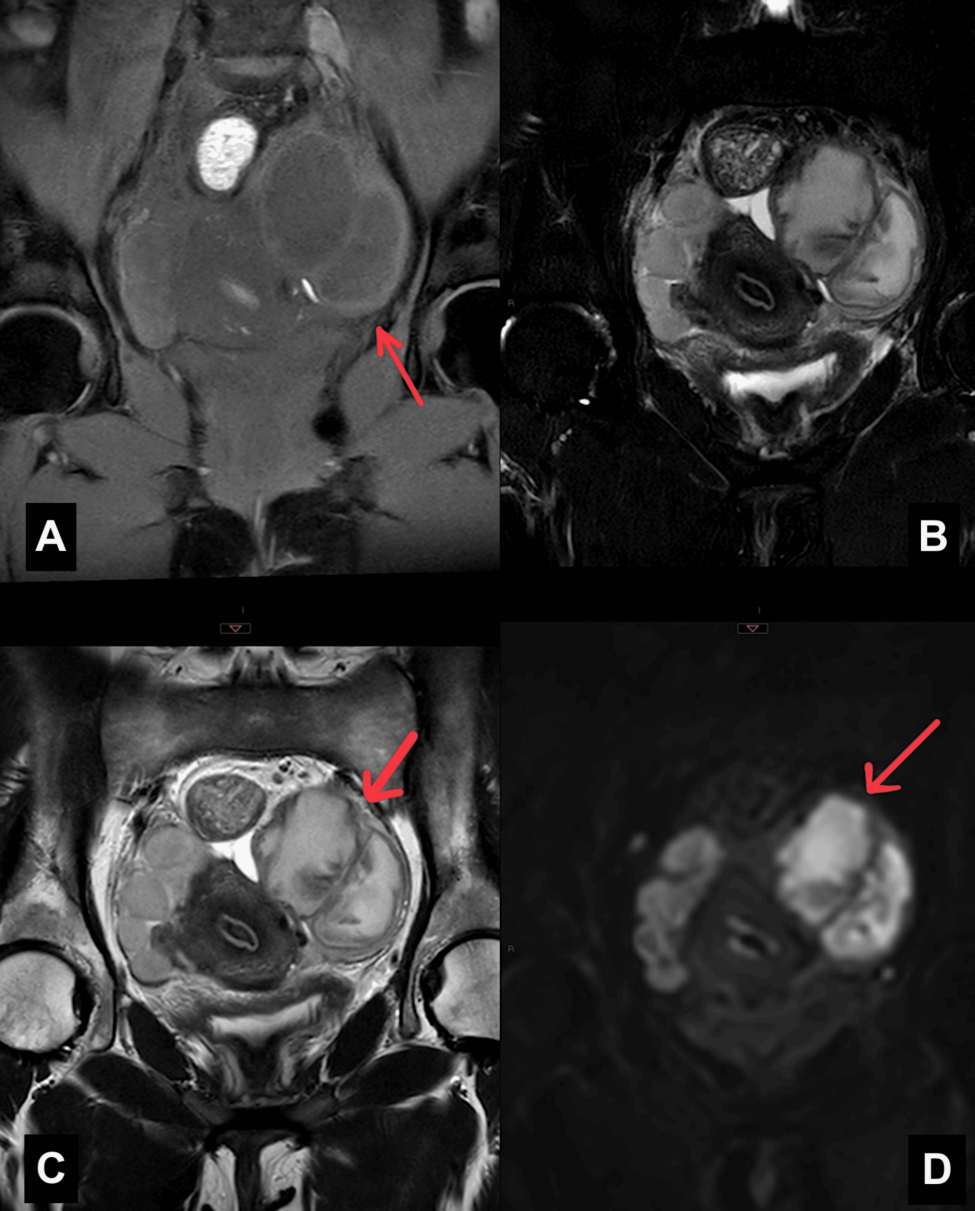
(A) T1, (B) T1 fat-saturated, (C) T2, and (D) DWI images of the pelvis showing a hypointense signal on T1, an intermediate signal on T2, and restricted diffusion on DWI, suggestive of a TOA. T1: T1-weighted MRI image; T1 fat-saturated: T1 image with fat signal suppressed to highlight lesions; T2: T2-weighted MRI image; DWI: diffusion-weighted imaging; TOA: tubo-ovarian abscess
